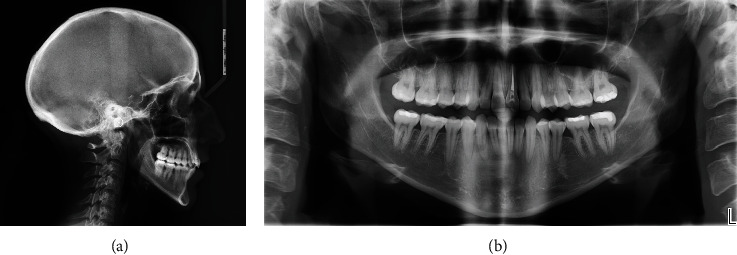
(a, b) Final X-ray records.
